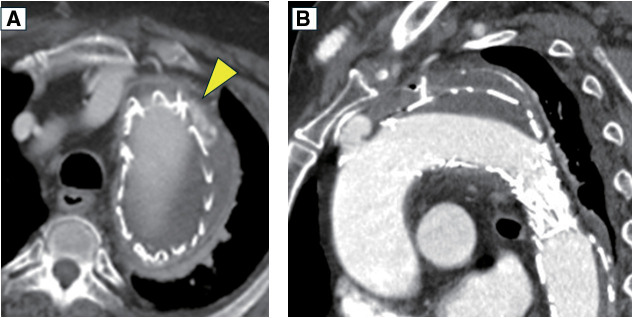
(A) Computed tomography scan findings (yellow arrowhead) showing minor type Ia endoleak. (B) Computed tomography scan findings raising suspicion of an infected thoracic aortic aneurysm.
